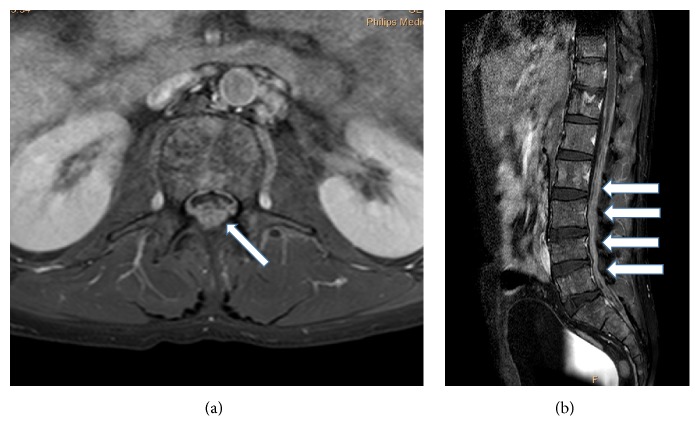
Gadolinium enhanced T1-weighted MRI images of leptomeningeal myeloma of the lumbosacral spine. (a) Axial and (b) sagittal image; arrows: contrast-enhanced myelomatous deposits (patient 11).
